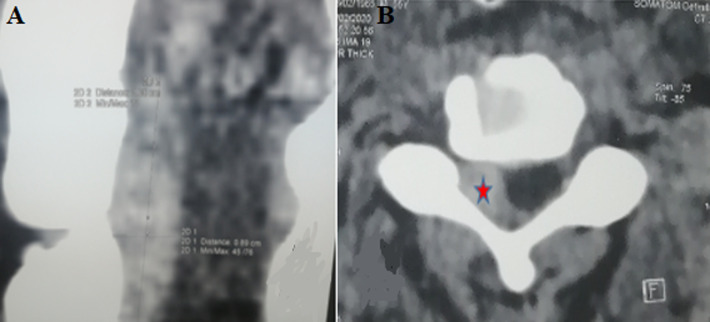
A) CT scan sagittal showed cervical epidural hematoma, extending from C3 to C5; B) CT scan axial view showing hematoma (red stars) compressing the spinal cord on the right side without associated bone lesion
